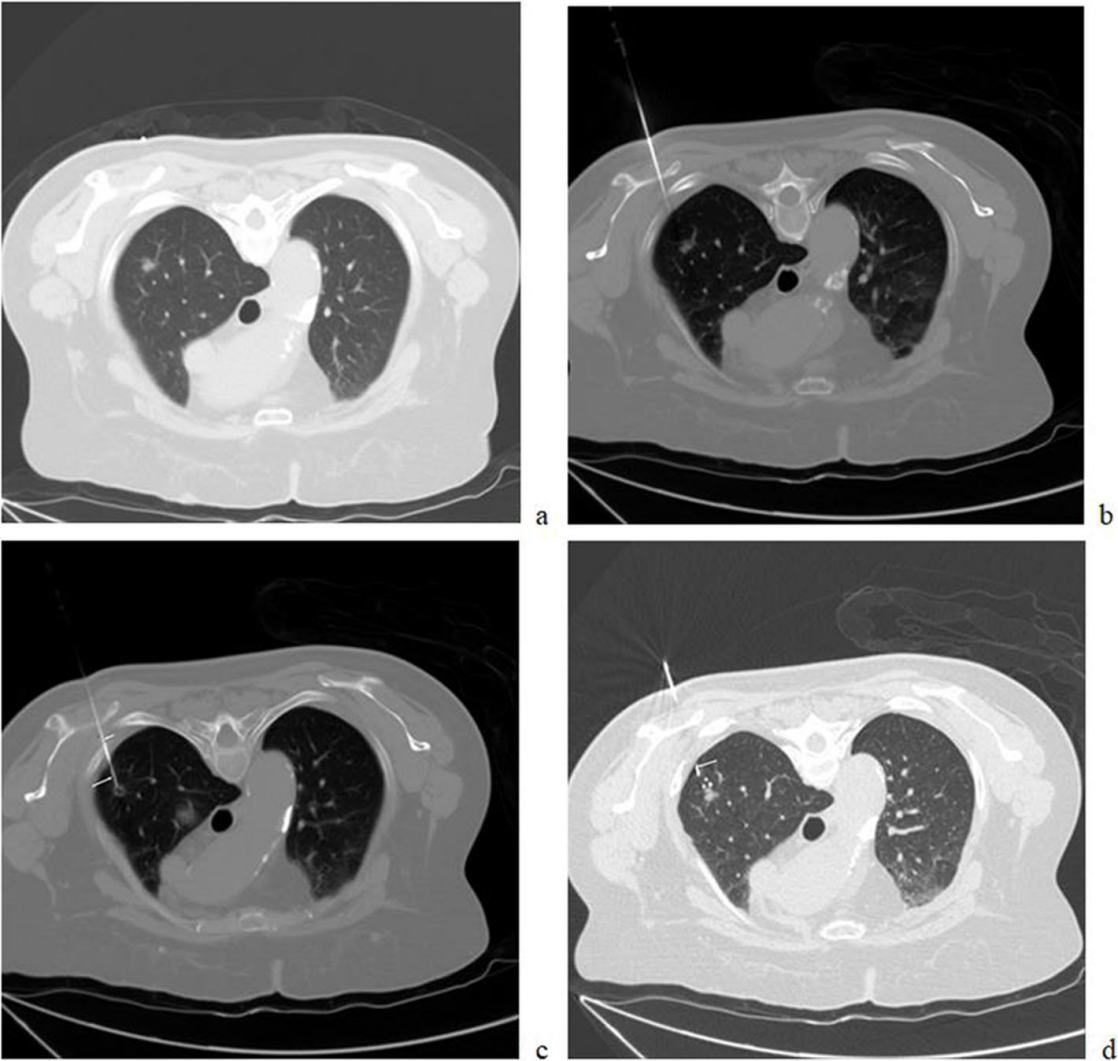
(a) Preoperative CT indicating a SBPN located at the right upper lobe; (b) A 17G needle was used to puncture the scapular; (c) An 18G needle (long arrow) was inserted into the lung for coil localization via the 17G needle (short arrow); (d) The coil tail (arrow) remained above the visceral pleura
